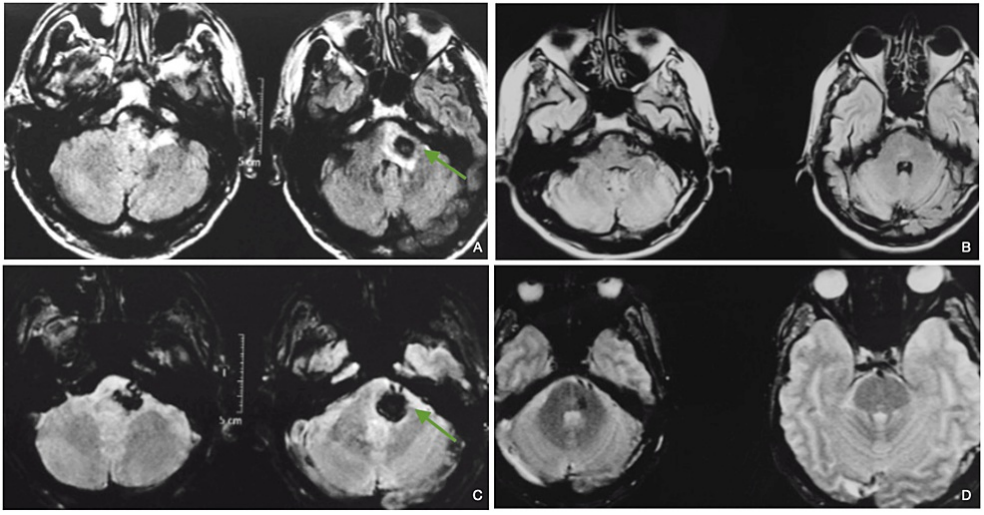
Pre and postoperative brain MRI routine delayed follow-up A and C preoperative T2WI and SWI, respectively, show a left intrapontine lesion consistent with ruptured cavernous malformation; B and D one-year follow-up T2WI and SWI, respectively, show satisfactory delayed postoperative control MRI. Green arrows denote the location of the intrapontine lesion and the approach trajectory in retrosigmoid access.
